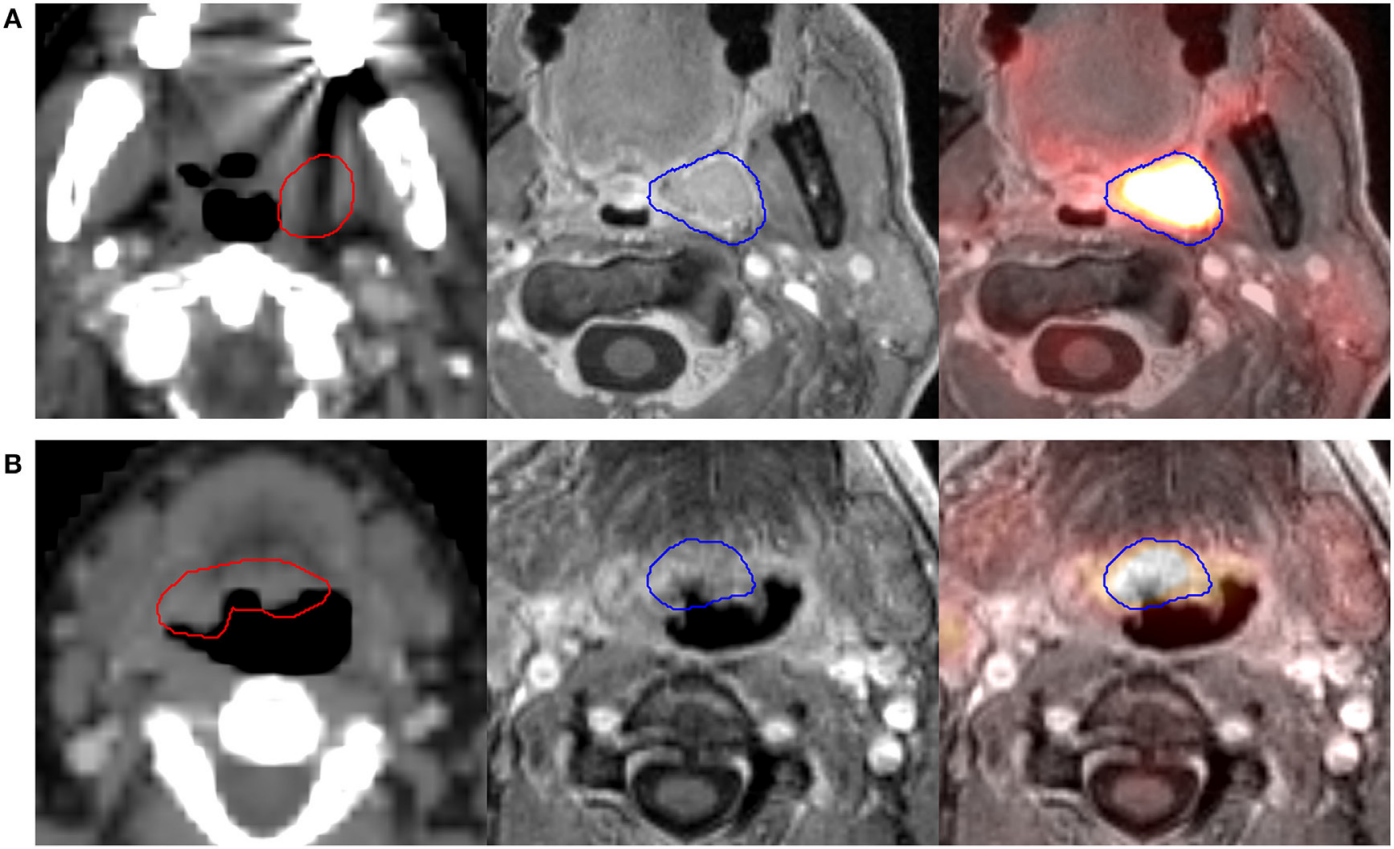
Two different patients [(A), patient 7, and (B), patient 11] where PET/MRI substantially altered the primary GTV. Scans from left to right are the planning computed tomography (CT) scan (GTV-CT shown in red), magnetic resonance imaging (MRI) component of PET/MRI, and fused PET/MRI (GTV-PET/MRI shown in blue). Patient 7 (A) had a left tonsil primary. PET/MRI showed soft tissue encroachment to the uvula not delineated on CT, with a resultant *increase* in GTV size. Patient 11 (B) had a base of tongue primary. Though the planning CT-GTV included indeterminate soft tissue in the right base of tongue, PET/MRI showed tumor limited to the central base of tongue, with a resultant *decrease* in GTV size.
